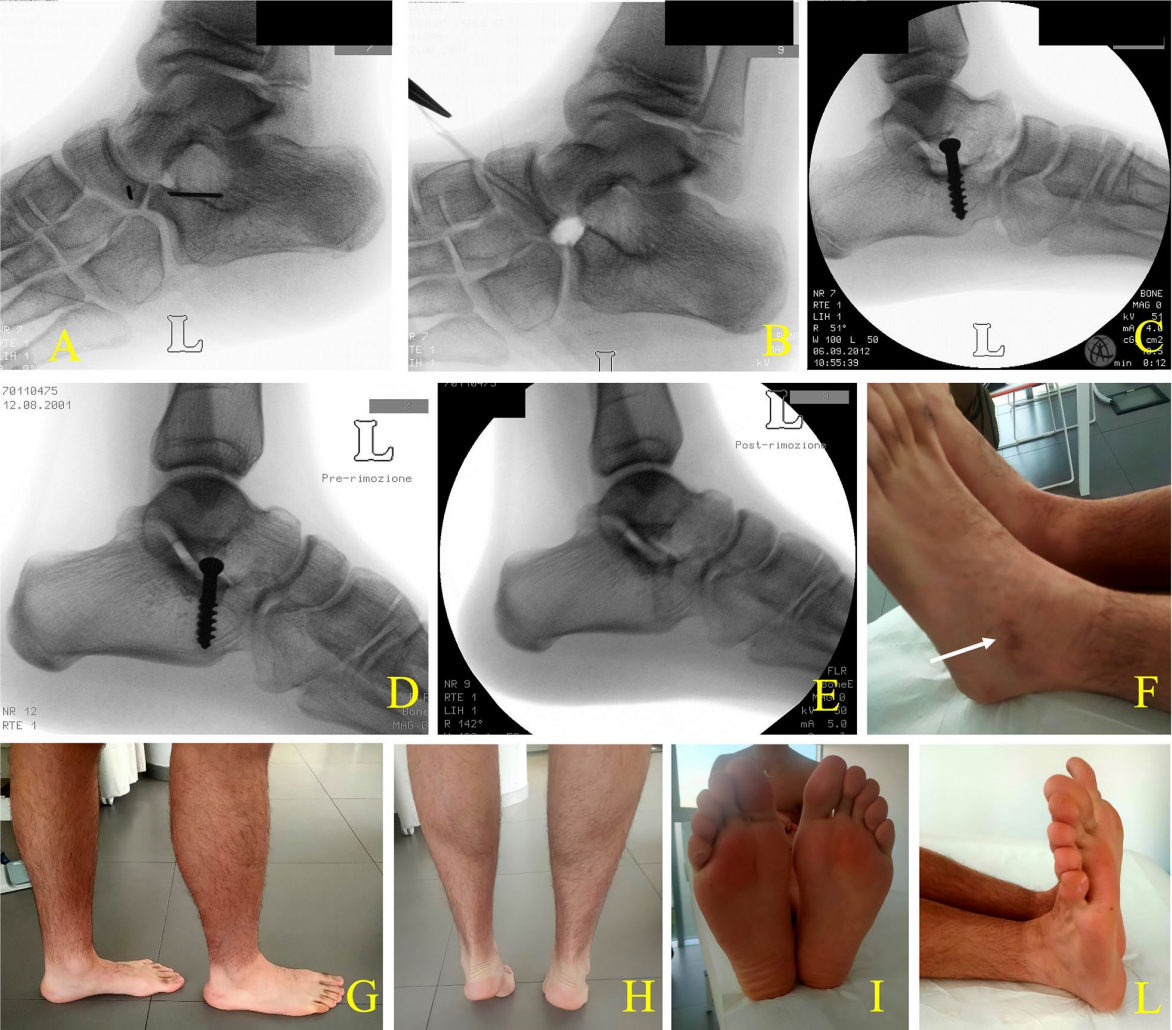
An 11-year-old boy affected by symptomatic rigid flatfoot deformity in CNC of the left foot. A Oblique x-ray projection shows the CNC. B Intraoperative radiographic evaluation after resection. C postoperative x-ray after subtalar arthroereisis with SESA technique for correction of the residual valgus deformity. D Preoperative x-ray before screw removal at 16 years and 10 months of age. E Postoperative x-ray after screw removal. F Skinscar (arrow) at follow-up at 23 years of age. G Medial arch at FU. H Functional outcome in tiptoes standing with normal pain-free range of motion and hindfoot alignment. I Plantar view. L Symmetrical ankle extension with right foot for comparison
